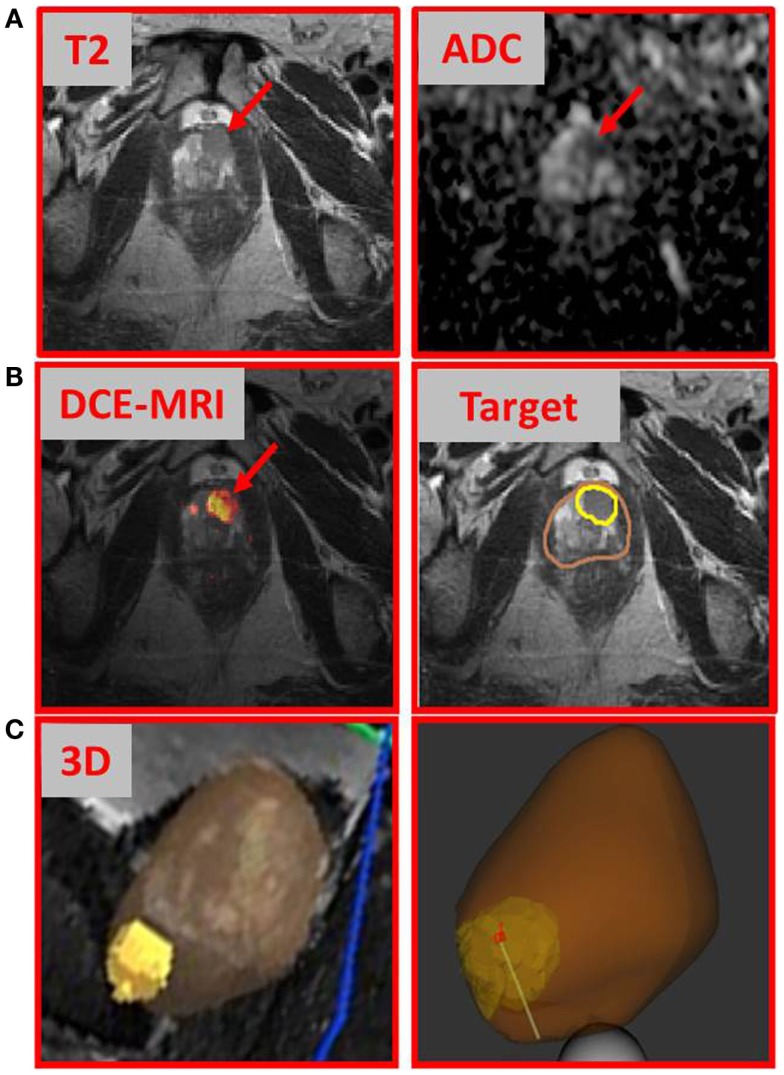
Multiparametric-MRI findings and directed prostate biopsy of the index lesion. (A) T2-weighted MRI and ADC map, with arrows indicating tumor area; (B) DCE-MRI intensity map with the lightest (yellow) part representing the areas of rapid contrast wash-in and gradual washout; and (C) tumor (yellow) and prostate (brown) contours. Both volumes were transferred to Artemis™ for fusion to the real-time ultrasound prior to prostate biopsy; 3D representation of the tumor with biopsy needle path shown.
